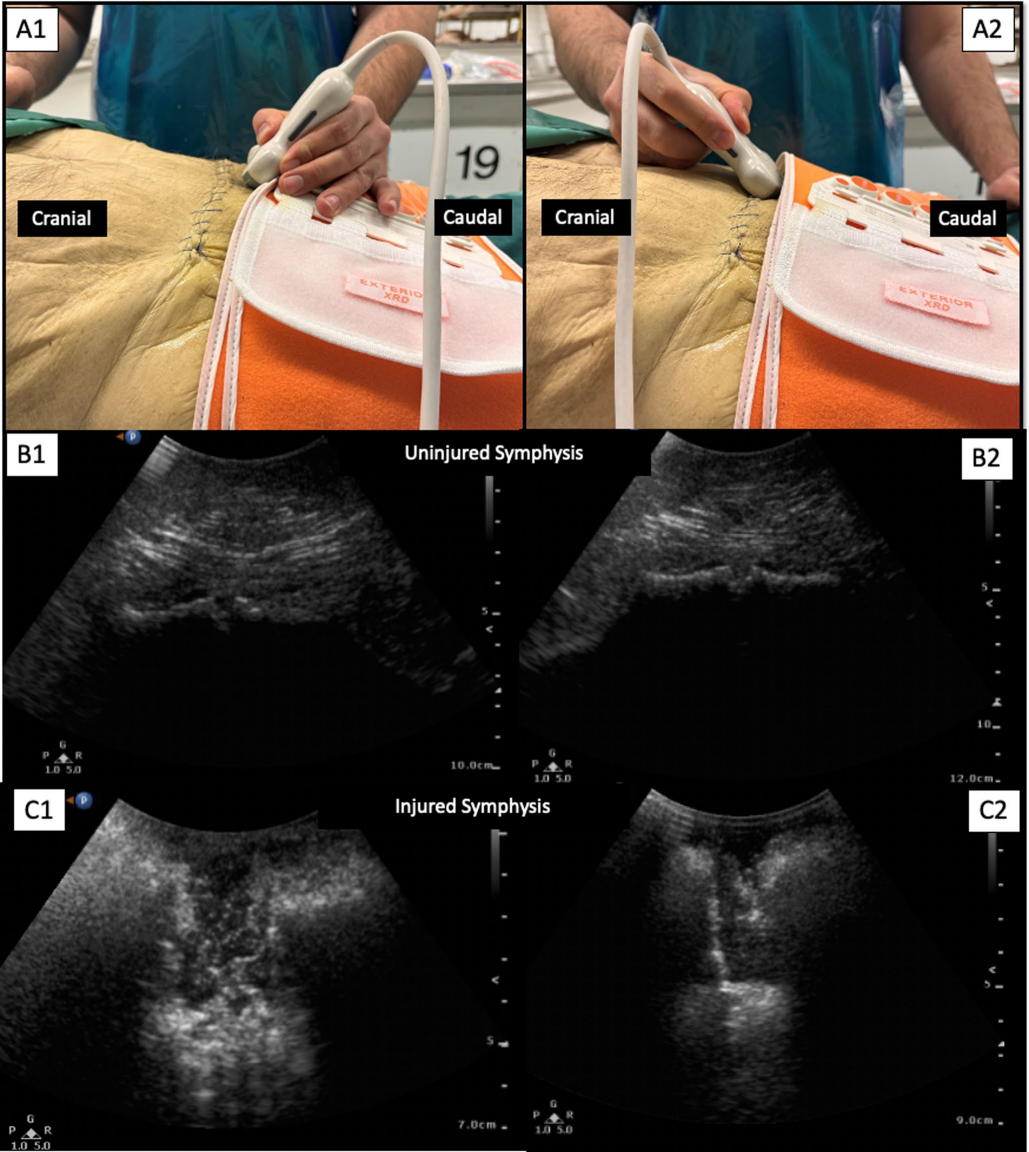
A: Clinical image of two different views on the symphysis. B: Ultrasound image of the uninjured symphysis (Control). C: Ultrasound image of the injured symphysis (here with diastasis of above2.5 cm)
